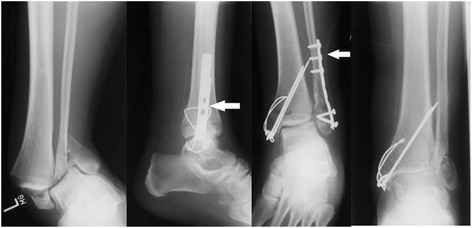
X-rays of an ankle fracture before and after ORIF with a drain left in place until osseous consolidation and following implant removal
